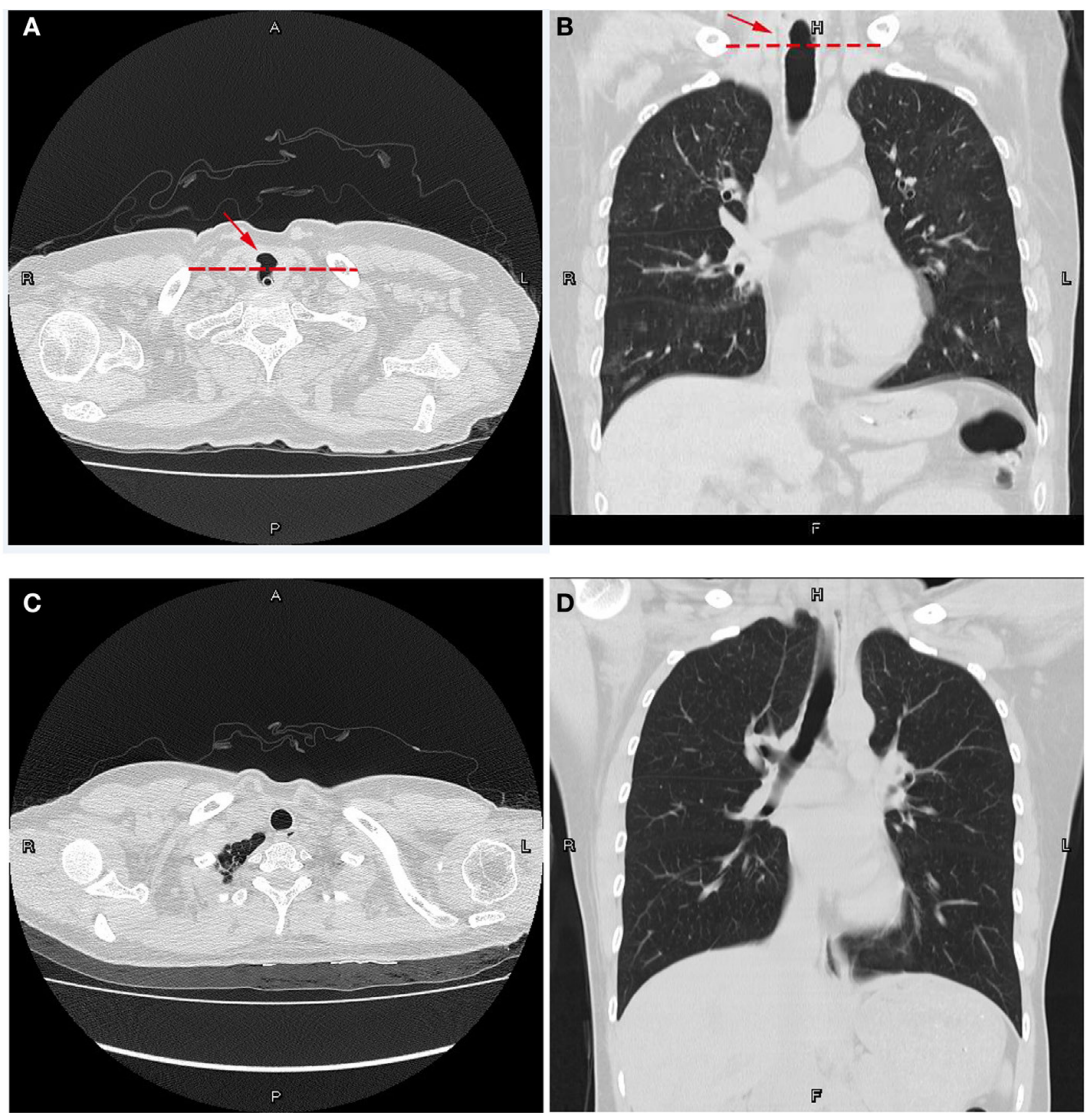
Computed tomography plain scans of the chest. (A,B) Preoperative views of the patient with a nasogastric tube in place. (A) Transverse position. (B) Coronal position at the posterior membranous part of the trachea. Note the cavity through the trachea and esophagus. Dotted lines represent the physiological interval. (C,D) Postoperative views. (C) Transverse position. (D) Coronal position. The tracheoesophageal fistula was repaired. The natural physiological interval lies across the trachea and esophagus, remodeling a tracheal cavity and an esophageal lumen.
